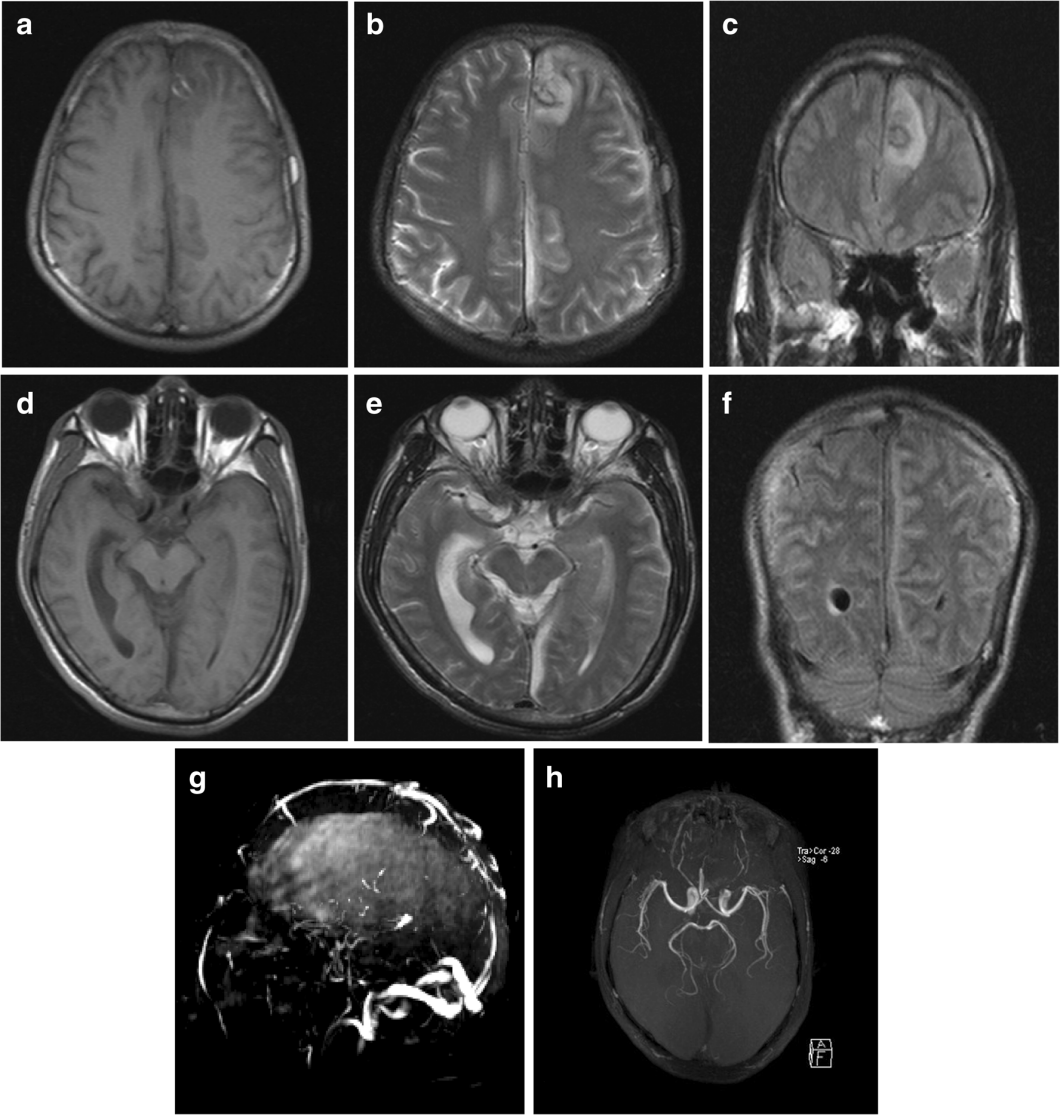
a-f Brain MRI revealed the left hemisphere swelled, whose cerebral sulcus and schizencephaly shallowed, especially in cerebral cortex, with patchy signal intensity which was gyriform on brain T2-weighted imaging (T2WI) and slightly high by fluid attenuation inversion recovery (FLAIR); the left frontal lobe, whose internal annular signal intensity was high on T1WI, low on T2WI and low on FLAIR was observed with patchy signal intensity that was slightly low on T1-weighted images (T1WI), slightly high on T2WI and high on FLAIR; the left falx was observed with striped signal intensity that was low on T1WI, high on T2WI and low on FLAIR. g MRV showed that straight sinuses were not clearly seen and superior sagittal sinus was not clear enough to be seen with rough cerebrovascular walls and focal luminal stenosis. h The cranial MRA showed no obvious abnormalities
